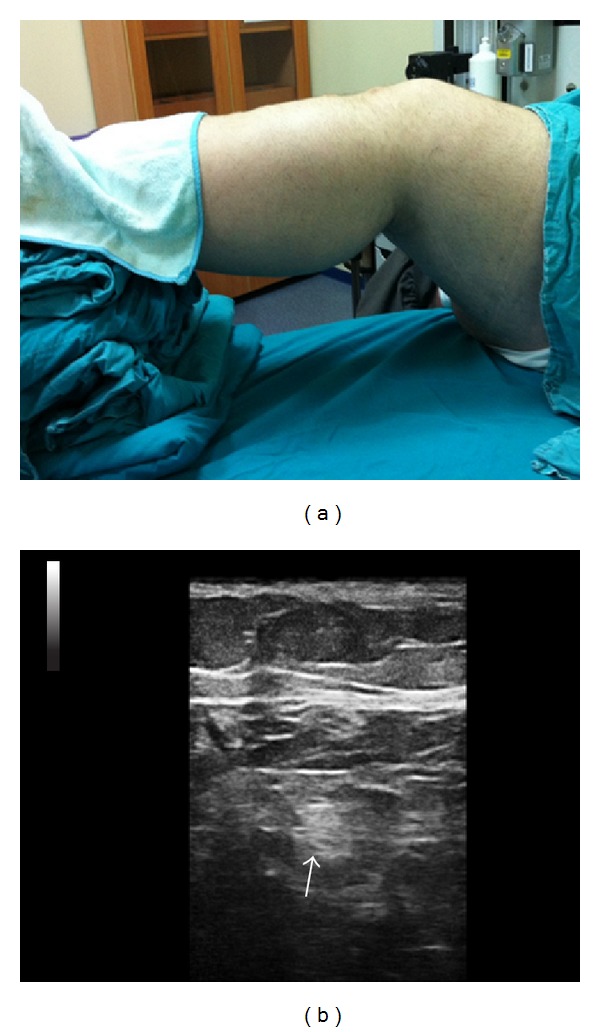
(a) Positioning for popliteal approach to the tibial nerve block. (b) Transverse sonogram in the popliteal region showing the tibial nerve as a hyperechoic nodule (arrow).
